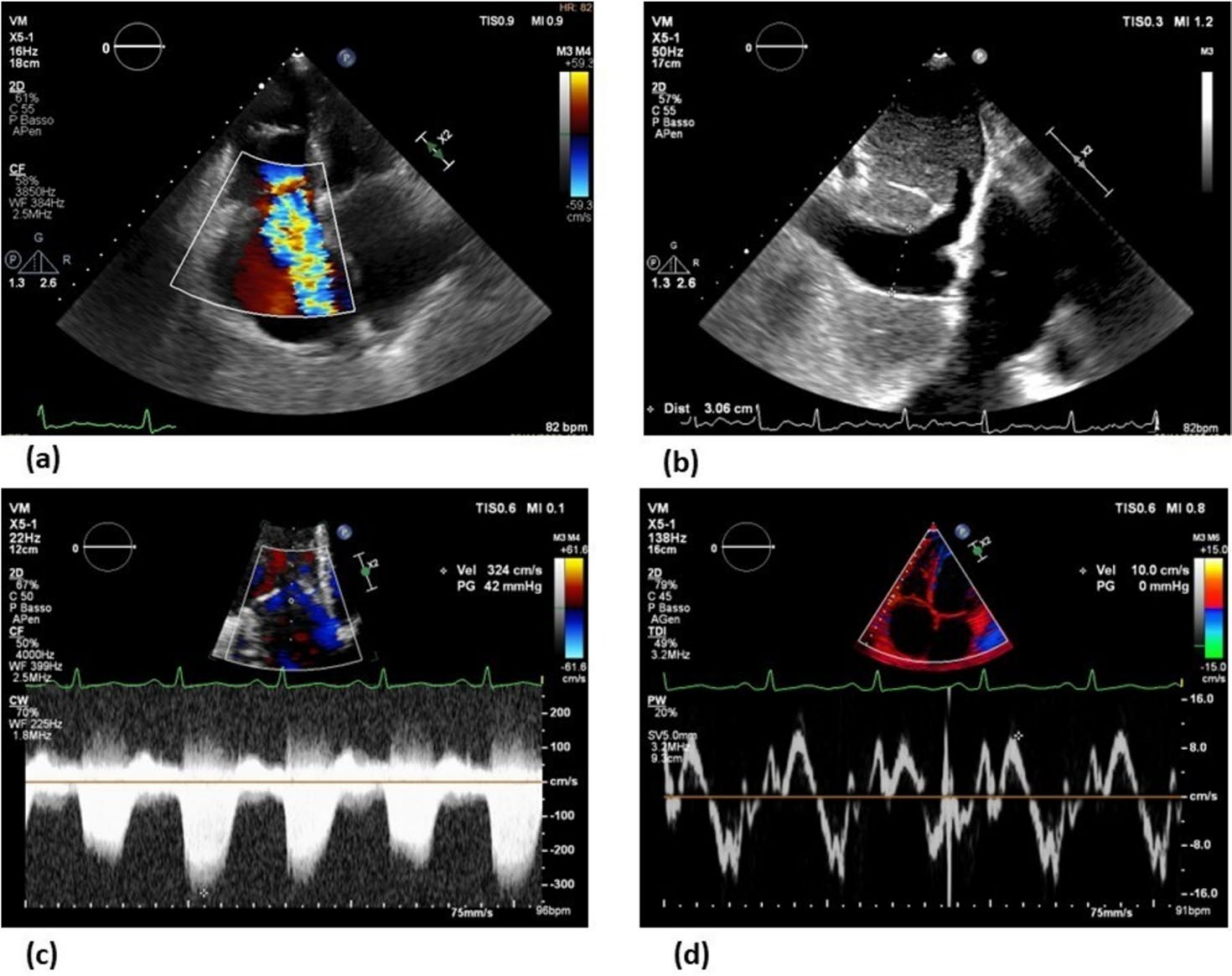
Echocardiographic evaluation of the volume status in heart failure (HF). A case of a patient with HF and fluid overload at the echocardiographic examination, requiring a prompt diuretic treatment. a The bidimensional assessment in the four-chamber view shows a dilated right ventricle, with a severe tricuspid regurgitation and an enlarged right atrium. b The subcostal view displays a marked dilation of the inferior vena cava with a reduced inspiratory collapse. c The continuous wave Doppler on the tricuspid regurgitations may allow an estimation of pressures in the right heart chambers. d Peak systolic velocity on tricuspid annulus by tissue Doppler imaging (S′ wave) represents a useful tool to assess the systolic function of the right ventricle
